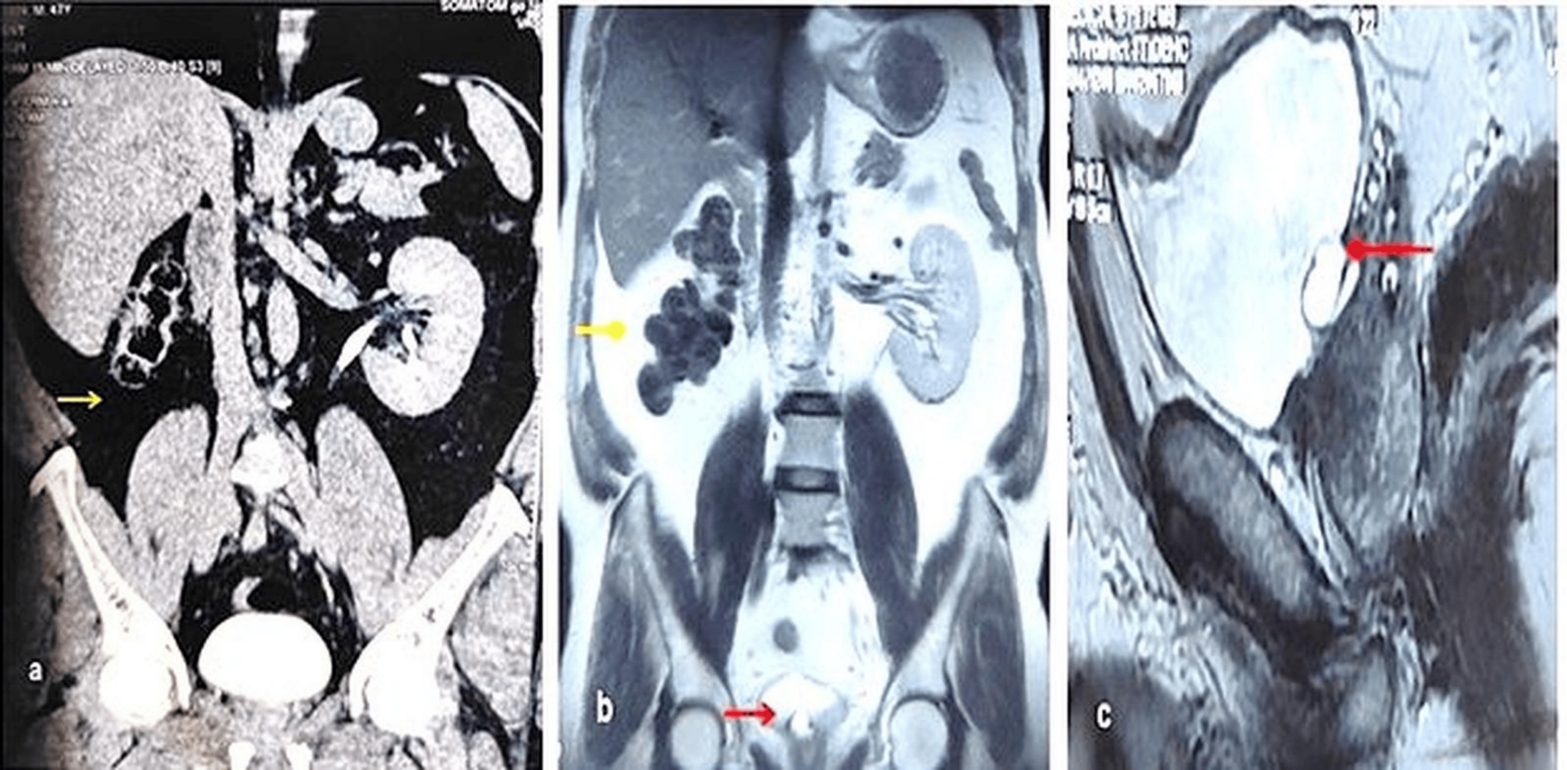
CT urography and MRI findings (a) CT urography findings showing an empty right renal fossa (yellow arrow). (b and c) Right renal agenesis (yellow arrow) with a right ureterocele (red arrow) CT: computed tomography; MRI: magnetic resonance imaging
